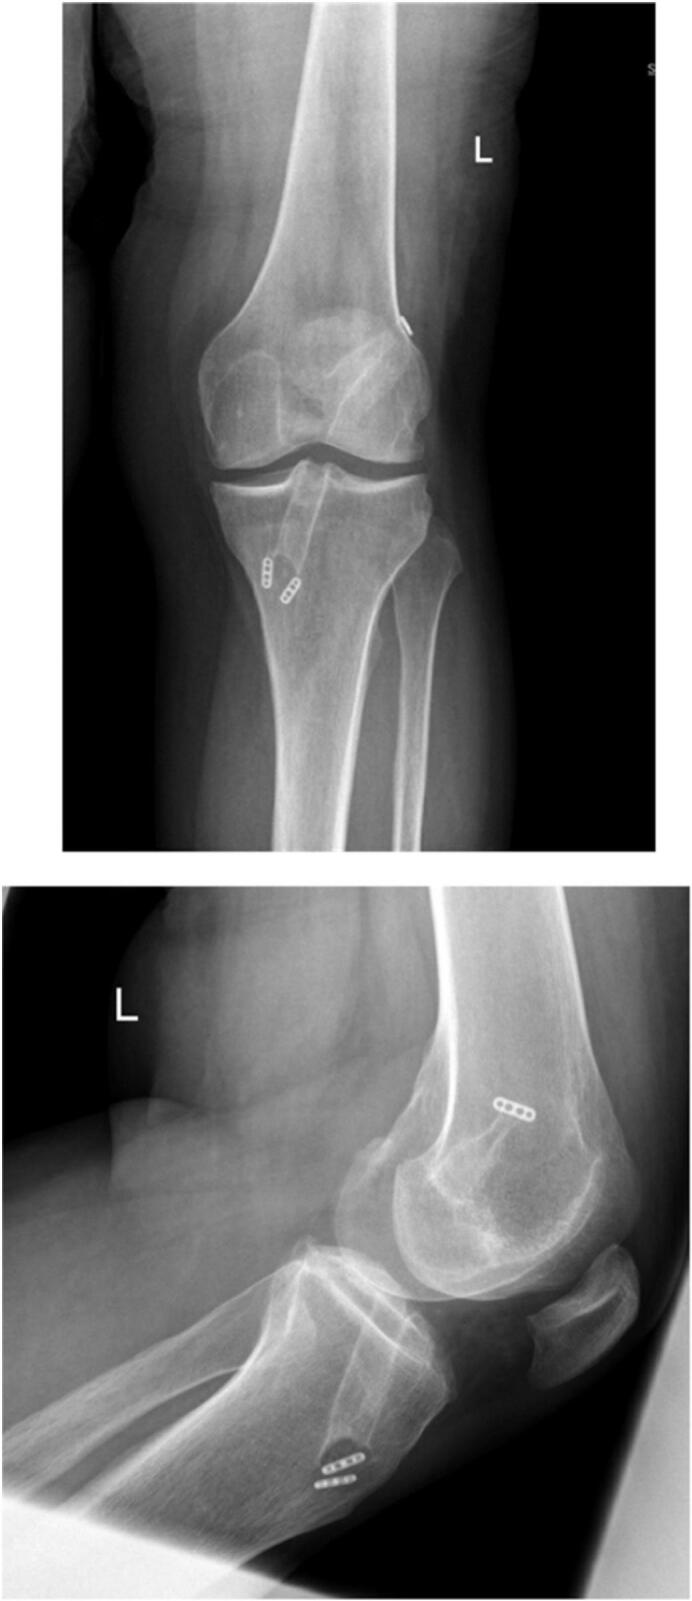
Full weight bearing radiograph of the left knee 2 years post-operatively.
